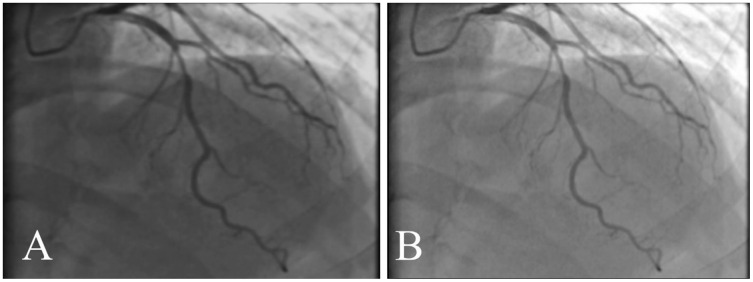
(A) X-ray image with noise. (B) X-ray image without noise.
